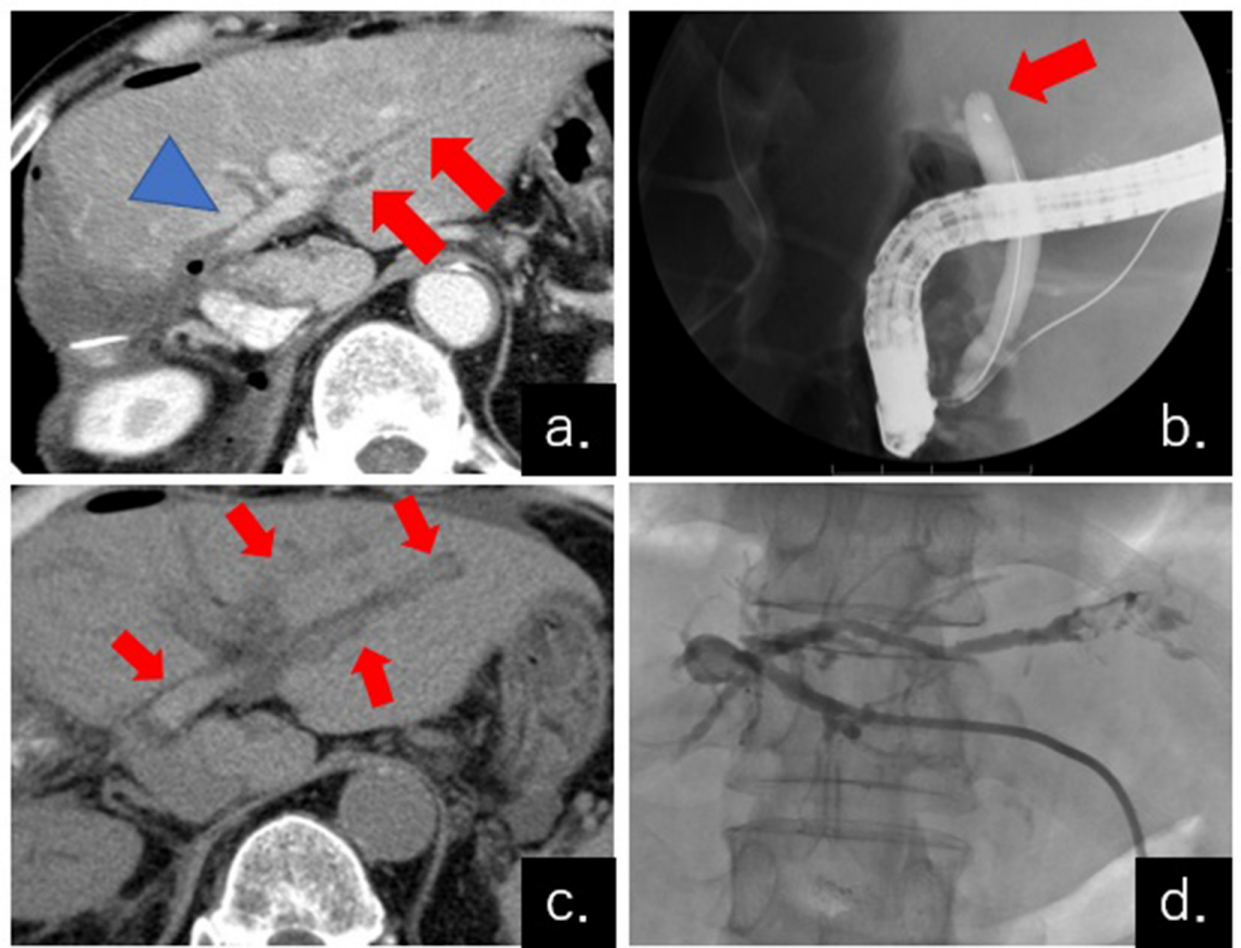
CT and fluorography of the liver on POD 2 to 5. (a) Slight dilation of the intrahepatic bile duct was confirmed on POD 2 (arrows). No PVT was displayed at this time (arrowhead). (b) Endoscopic retrograde cholangiopancreatography was performed on POD 3. However, the guidewire could not be cannulated into the upper side of the common bile duct (arrow), which indicated an obstruction. (c) PVT was detected incidentally during the PTBD procedure on POD 5. The intrahepatic portal vein looked highly absorbed in the plain CT (arrows), which indicated a fresh thrombus. (d) Although PTBD was performed, the guidewire could not be cannulated. POD, post-operative day; PTBD, percutaneous transhepatic biliary drainage; PVT, portal vein thrombosis.
